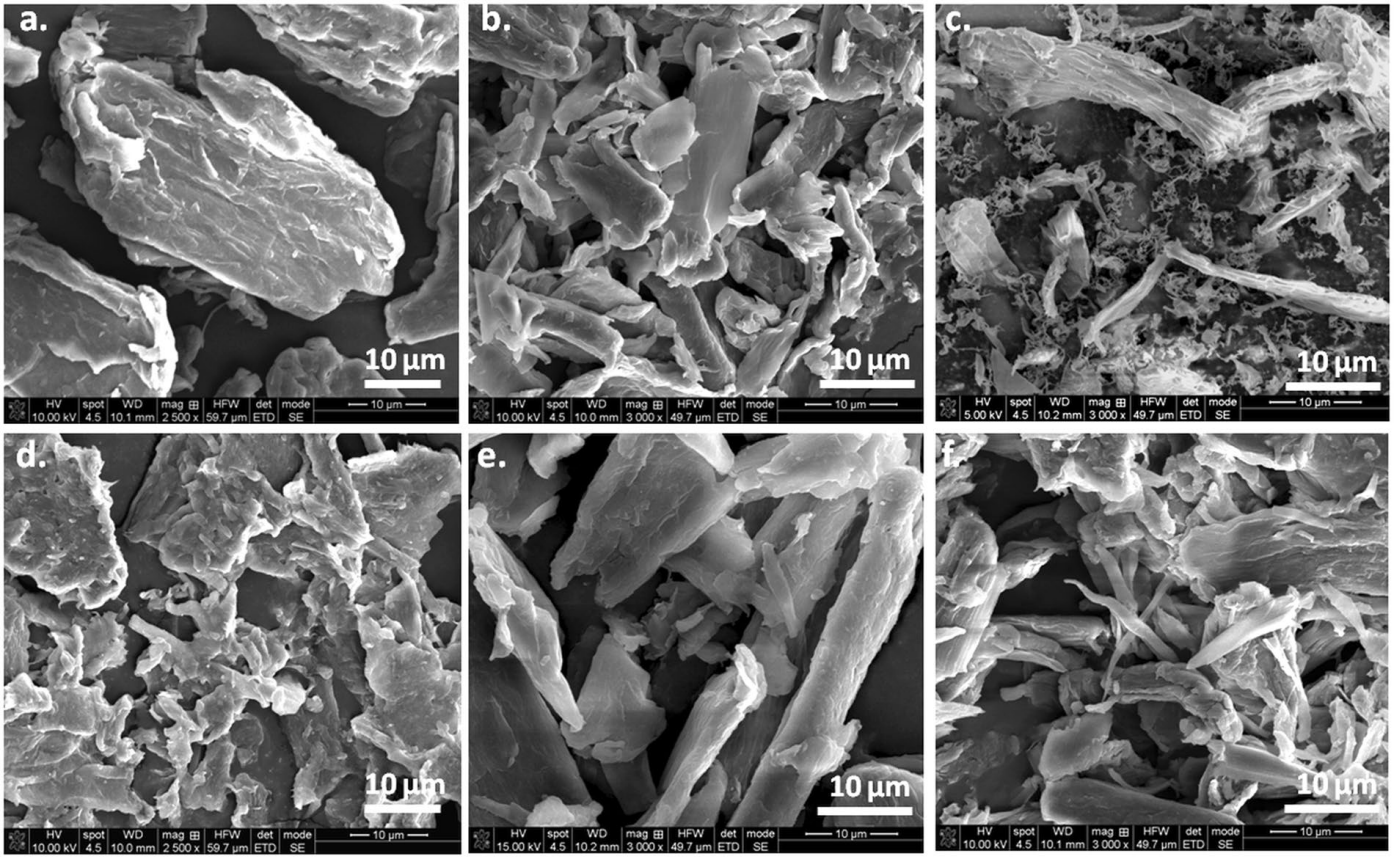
SEM images of untreated MCC (a), ultrasonicated MCC (b), and plasma treated MCC in Ar (c) Ar/N_2_ (d) Ar/O_2_ (e) and Ar-ACN (f).
